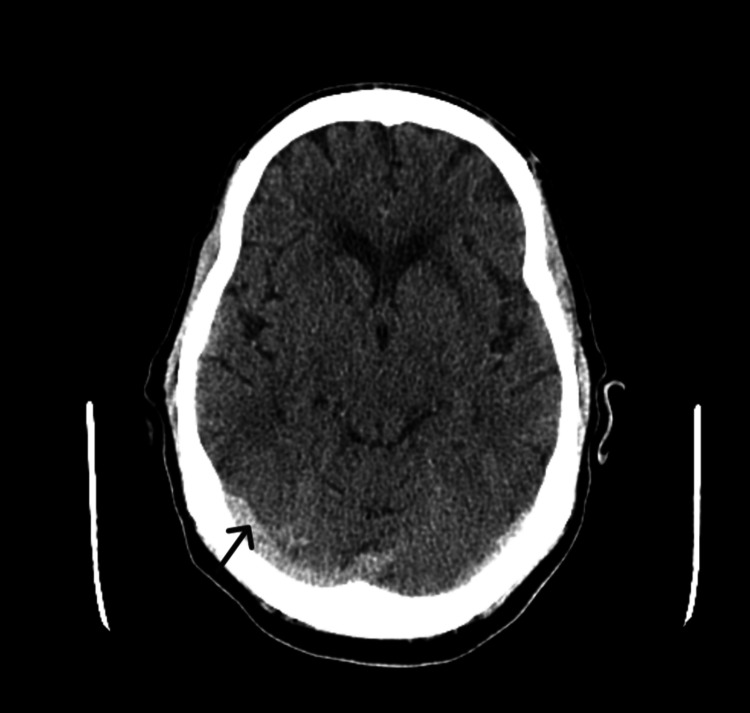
CT of the head without contrast showing right parietal paramedian extra-axial density suspicious for either hemorrhage or neoplasm (arrow)
